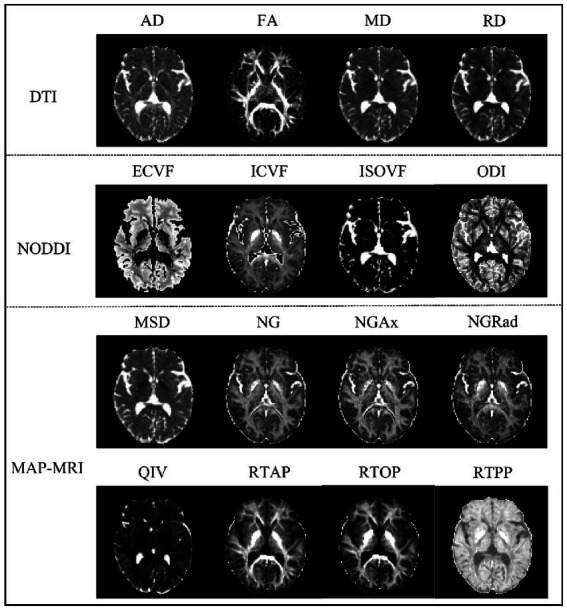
Comparison of three diffusion MRI maps showing brain microstructure. The top panel shows DTI parameters with Axial Diffusivity (AD), Fractional Anisotropy (FA), Mean Diffusivity (MD), and Radial Diffusivity (RD). The middle panel presents NODDI parameters: Extracellular Volume Fraction (ECVF), Intracellular Volume Fraction (ICVF), Isotropic Volume Fraction (ISOVF), and Orientation Dispersion Index (ODI). The bottom panel displays MAP-MRI parameters: Mean Squared Displacement (MSD), Non-Gaussianity (NG), Axial Non-Gaussianity (NGAx), Radial Non-Gaussianity (NGRad), Q-space Inverse Variance (QIV), Return-to-Axis Probability (RTAP), Return-to-Origin Probability (RTOP), and Return-to-Plane Probability (RTPP). Each parameter map reveals specific aspects of brain tissue microstructure.
